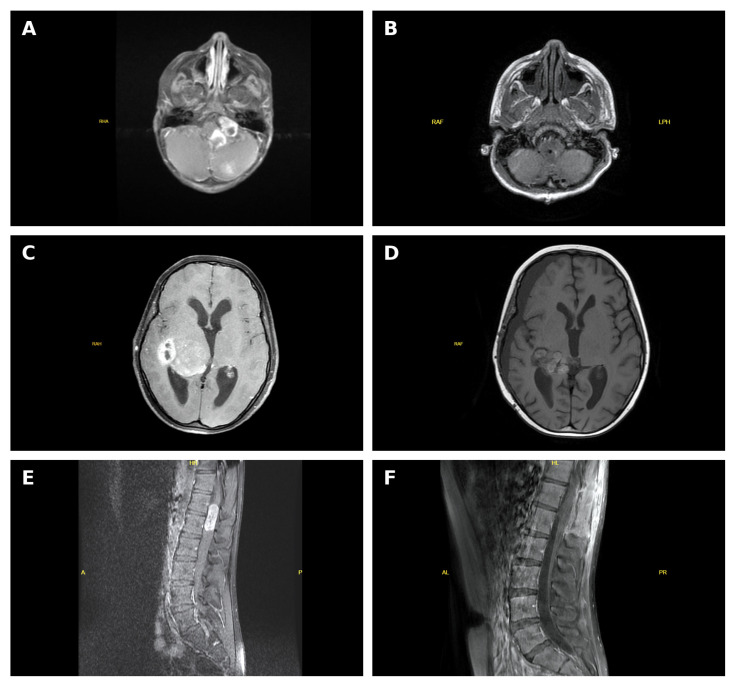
Representative magnetic resonance imaging findings of pediatric ependymoma according to tumor location. (A,B) Posterior fossa ependymoma. Preoperative contrast-enhanced MRI demonstrates a posterior fossa mass, and postoperative imaging shows the resection cavity. (C,D) Supratentorial ependymoma. Preoperative axial MRI demonstrates a supratentorial lesion, while postoperative MRI shows postsurgical changes after tumor resection. (E,F) Spinal ependymoma. Preoperative sagittal MRI demonstrates an intradural spinal lesion, and postoperative MRI shows postoperative changes following resection.
